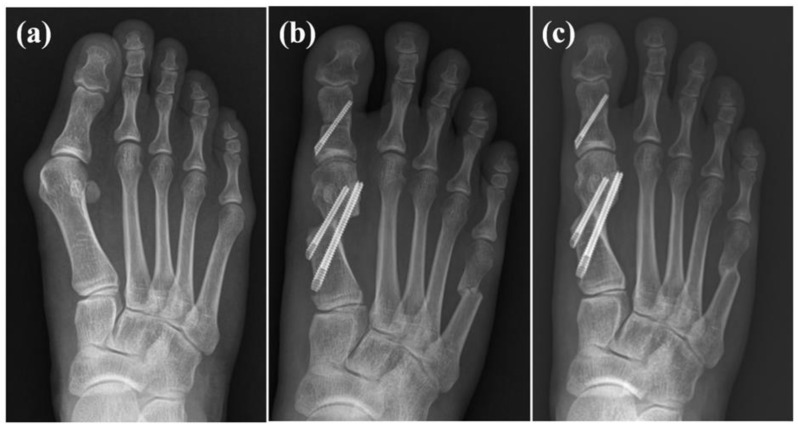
Case example of female, 35-year-old patient. (a) Preoperative radiographs show moderate hallux valgus deformity; (b) 6 weeks following surgery, bunionette correction was also performed simultaneously; (c) 2 years following surgery.
